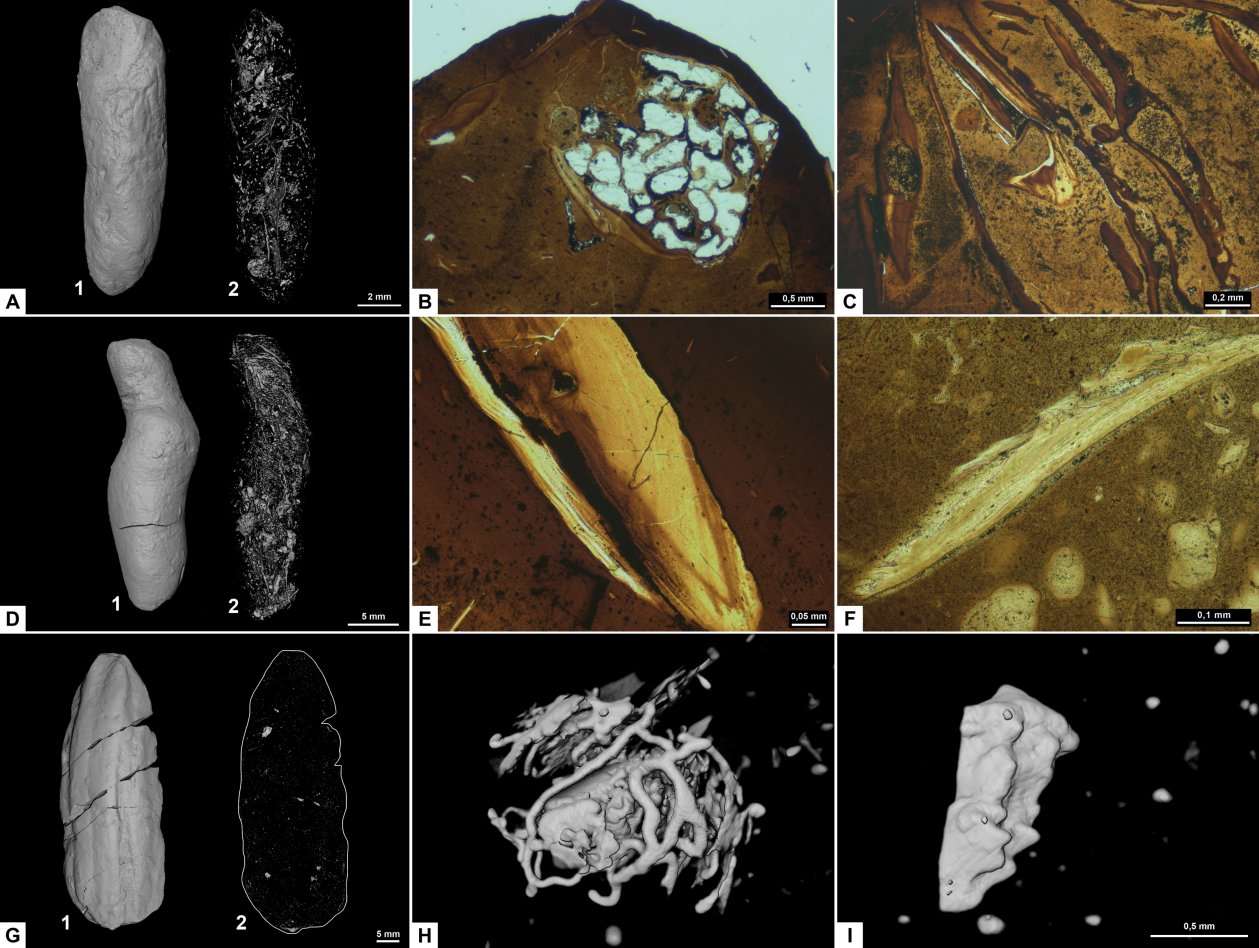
CT-scans (A, D, G–I) and thin sections (B–C, E–F) of morphotype A. (A1–A2) External and internal view of sub-morphotype A, PMO 250.270. (B) Tetrapod bone in thin section, PMO 250.004. (C) Fish vertebrae, PMO 250.009. (D1–D2) External and internal view of sub-morphotype A2, PMO 150.275. (E) Fish scale, PMO 249.999. (F) Degraded bone fragments, PMO 250.000. (G1–G2) External and internal view of sub-morphotype A3, PMO 250.273. (H) Burrows with a width of approximately 0.5 mm over a total area of three mm, PMO 250.530. (I) Conodont element measuring 1.22 mm in length, PMO 250.273.
